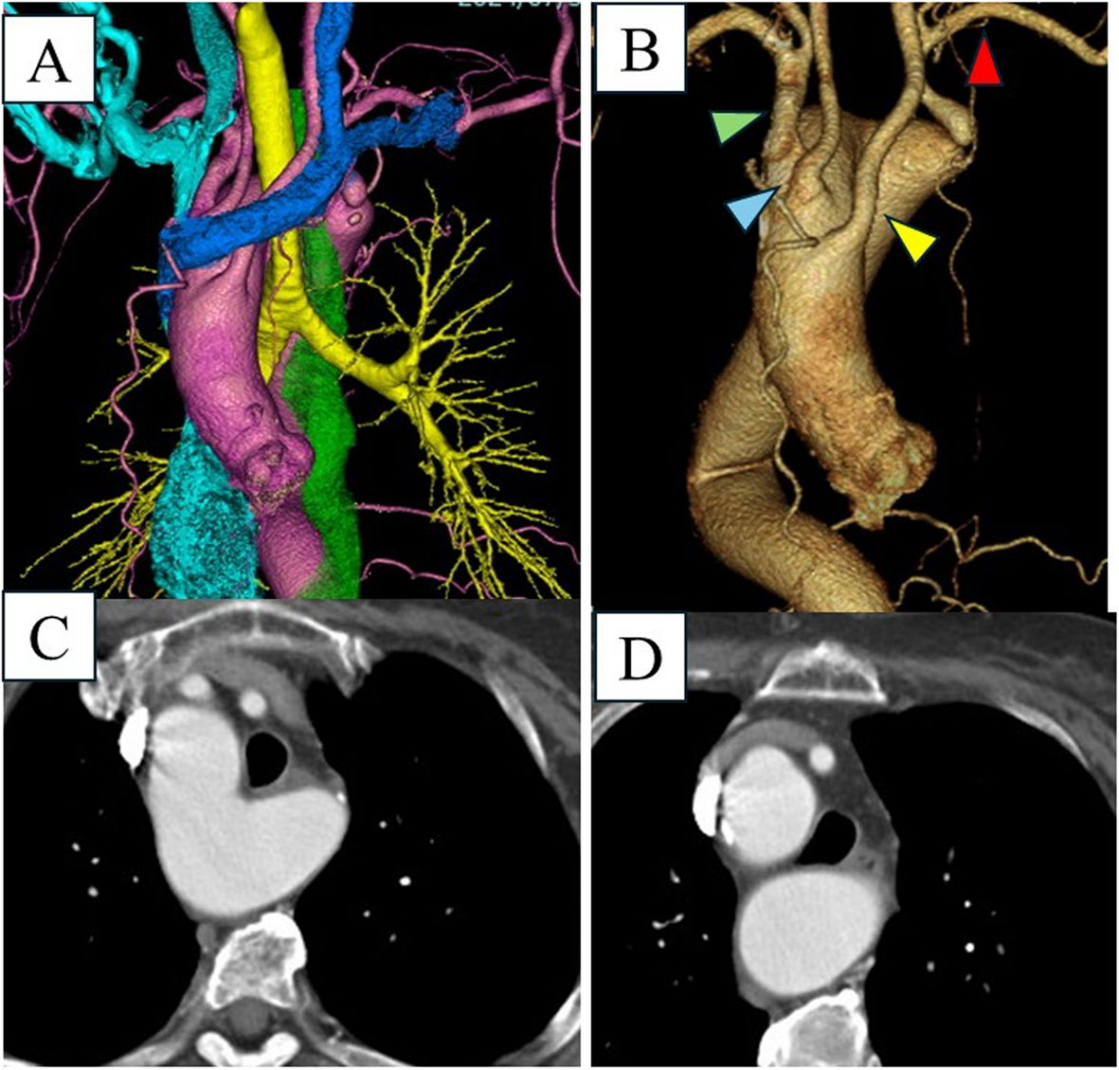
Preoperative computed tomography image. A Three-dimension computed tomography image. The blue structure represents the innominate vein, the yellow represents the trachea, the green represents the esophagus, and the red represents the aorta. B Three-dimension computed tomography image for aorta. The yellow arrowhead indicates the left common carotid artery, the blue arrowhead indicates the right common carotid artery, the green arrowhead indicates the right subclavian artery and the red arrowhead indicates the left subclavian artery. C Axial computed tomography image at the level of the Kommerell’s diverticulum. D Axial computed tomography image at the distal level of the Kommerell’s diverticulum
